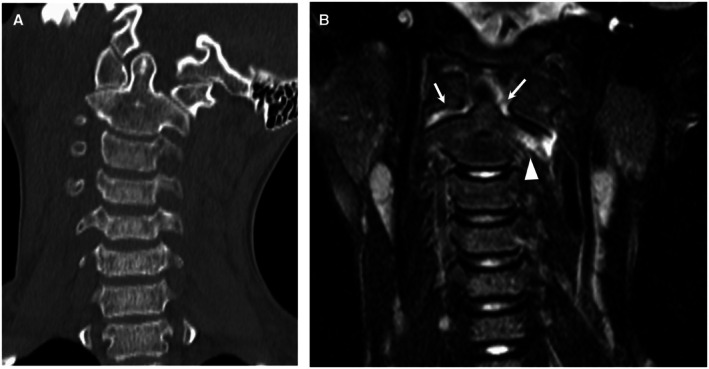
A 12‐year‐old girl with ANA‐positive JIA presenting with torticollis. (A) Coronal CT showed atlantoaxial rotatory subluxation. (B) Fat‐suppressed T2‐weighted coronal MRI showed atlantoaxial synovitis with increased signal (arrows) and bone marrow signal change in the left lateral mass of C2 (arrowhead).
